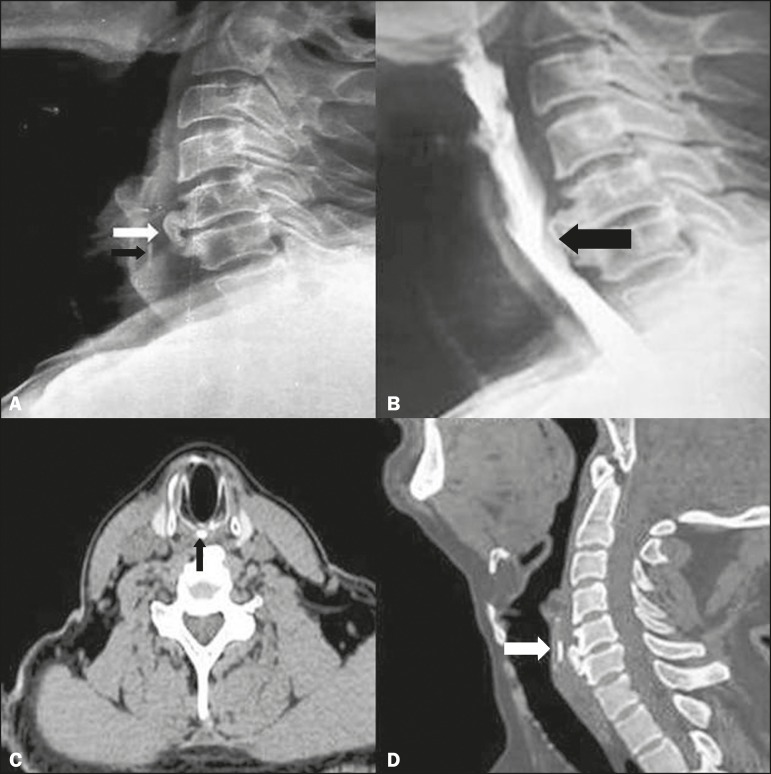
A: Soft tissue X-ray of the neck, in lateral view, showing large
